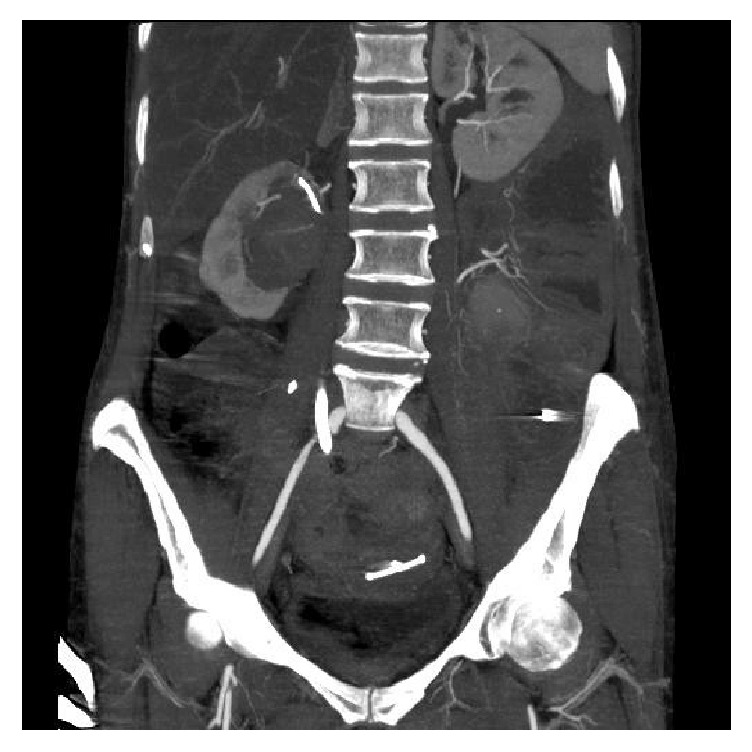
Abdominal-pelvic CT scan frontal cut passing through the right ureter: endoureteral balloon probe in place in the right ureter at the fistula with the right primary iliac artery.
